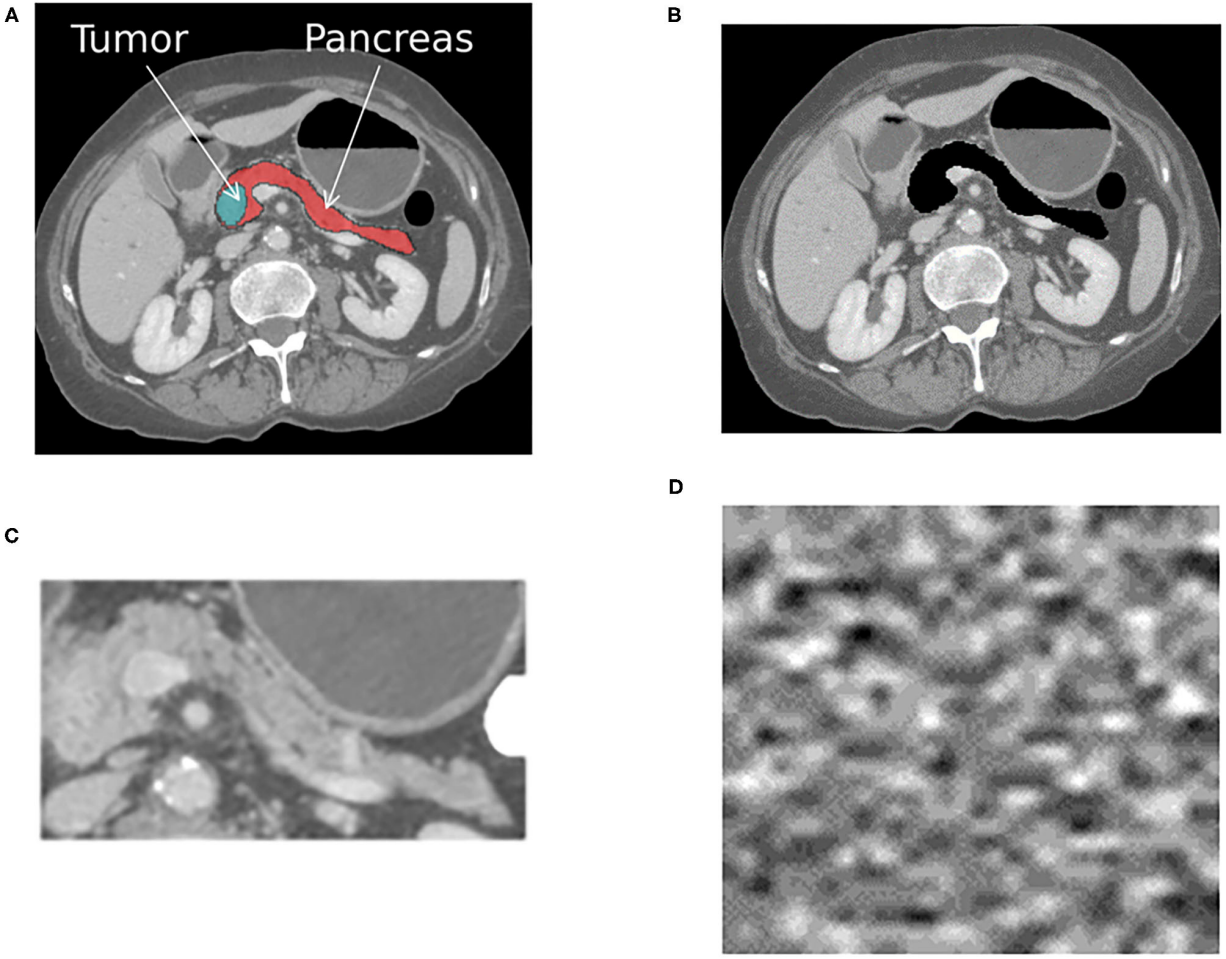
The figure shows an example cross-sectional slice from a single patient with pancreatic cancer that was processed into four different input formats, as shown. A different AI system was trained with each input format, resulting in four different systems that were then tested on each format. (A) The original image with the pancreas and tumor present. (B) The original image with the pancreas and tumor removed. (C) The anatomy surrounding the pancreas is cropped out, and only the pancreas and tumor remain. (D) Noise image generated from the patient CT scans. The same processing was applied to all CT scans within the positive for pancreatic cancer and normal pancreas classes.
